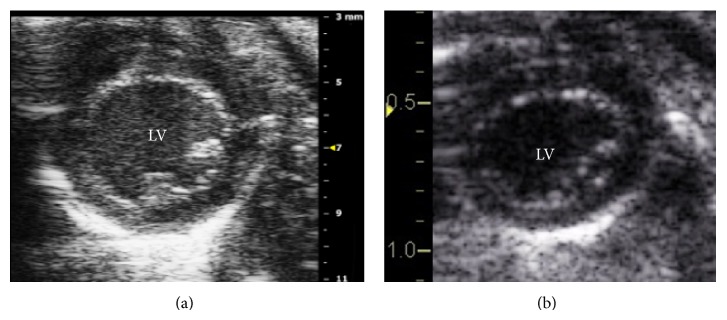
2D greyscale of parasternal short axis acquired by (a) VSI Vevo 2100 and (b) GE Vivid 7. LV: left ventricle.
